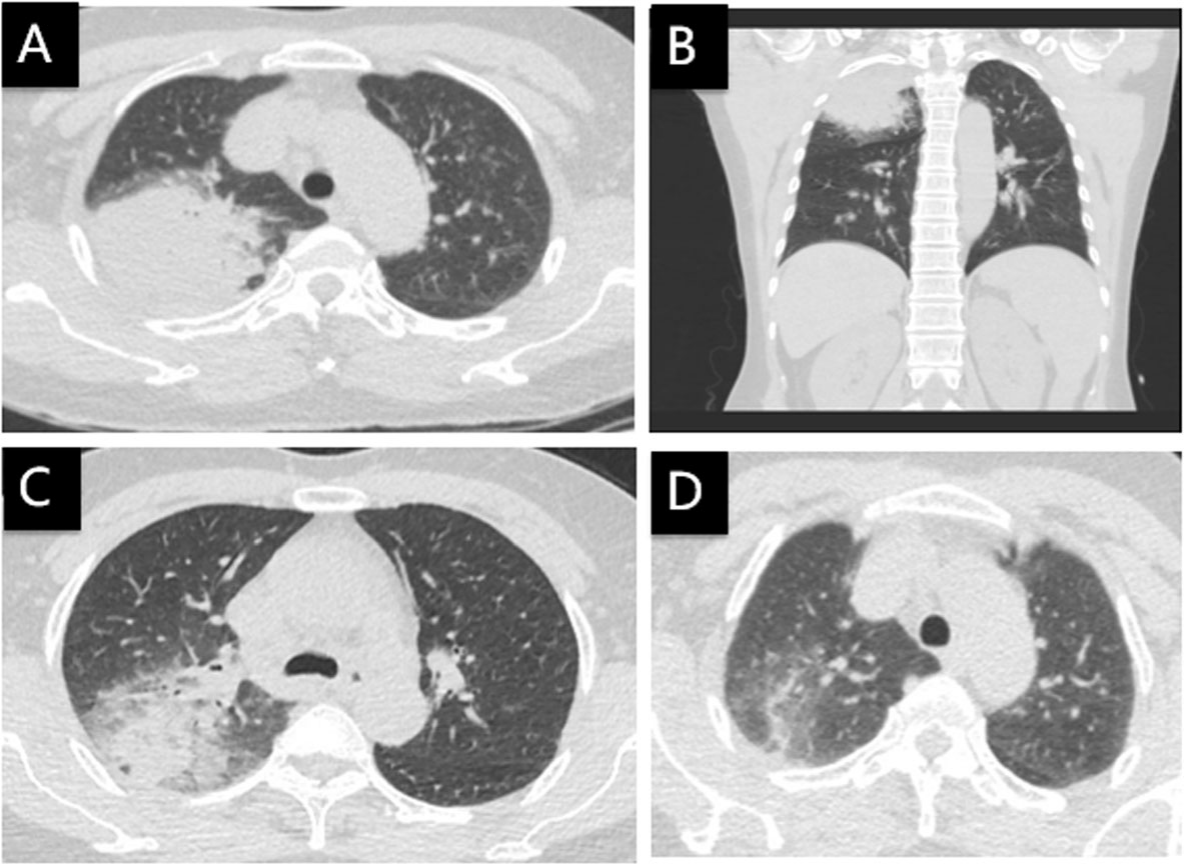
Chest CT of case 4: a, b On the day of admission, c Eight days after treatment with moxifloxacin, and dSixteen days after discontinuation
